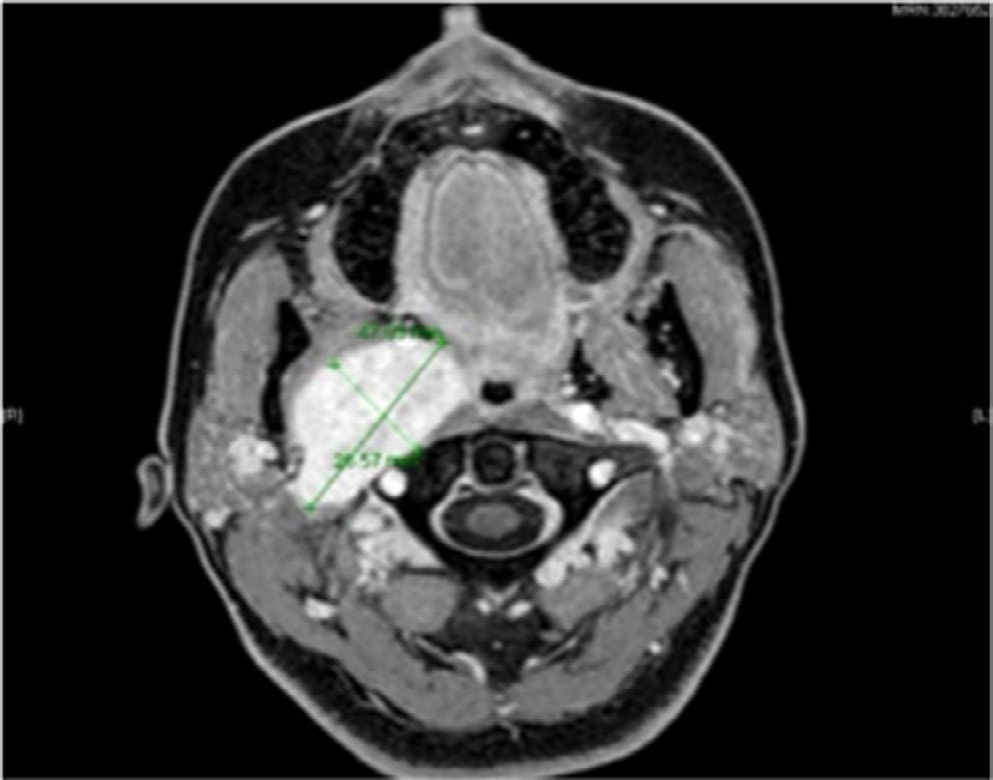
Showed a redemonstration of a T2 hyperintense, lobulated, well‐defined lesion within the right retro‐styloid parapharyngeal space measuring 4.2 × 2.5 × 4.5 cm but that has increased in comparison to the prior MRI. When it measured approximately 3.4 × 1.9 × 3.7 cm and measured similarly.
